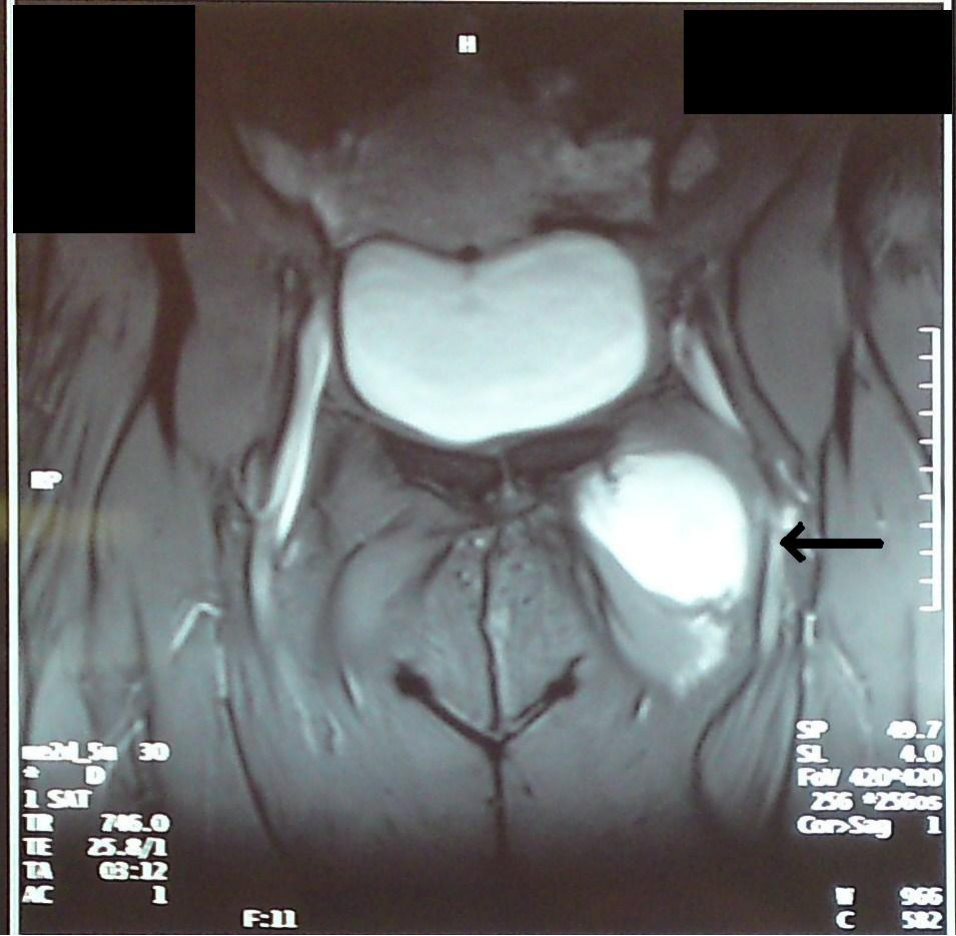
Coronal T2-weighted MRI of left groin Intramuscular myxoma.
